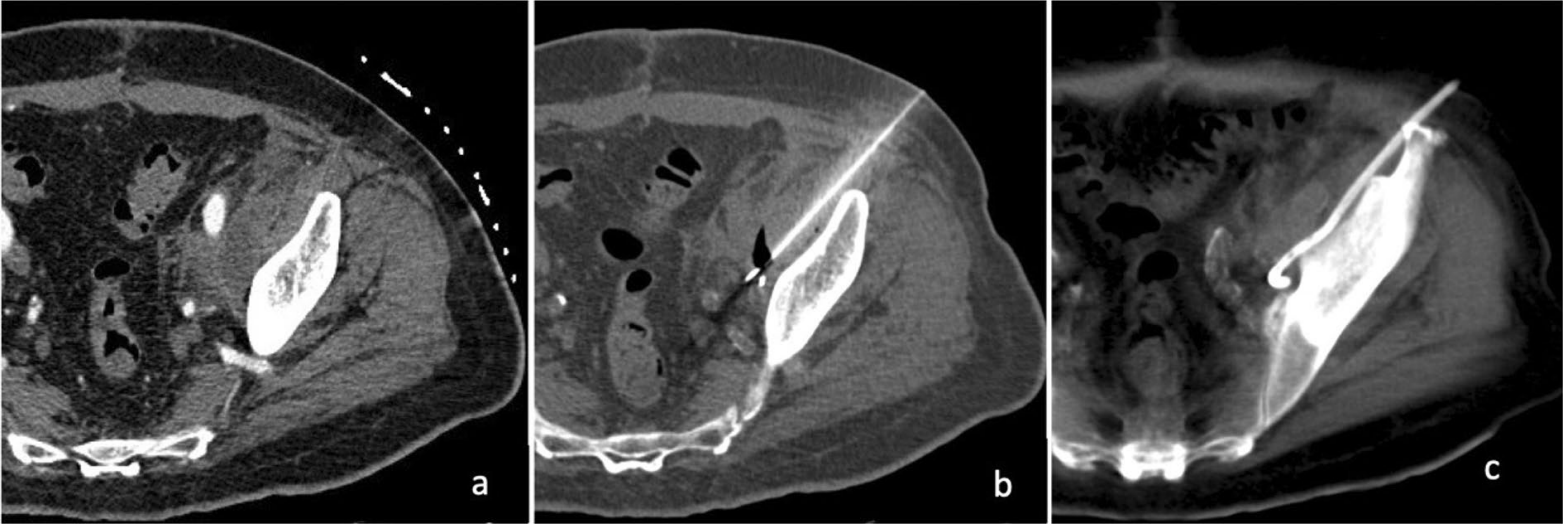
Left psoas abscess in a 76-year-old patient (a). The positioning of the guide wire (b) and the placement of a 12F PIGTAIL catheter (c) are carefully obtained under CT-guidance avoiding peritoneal cavity
